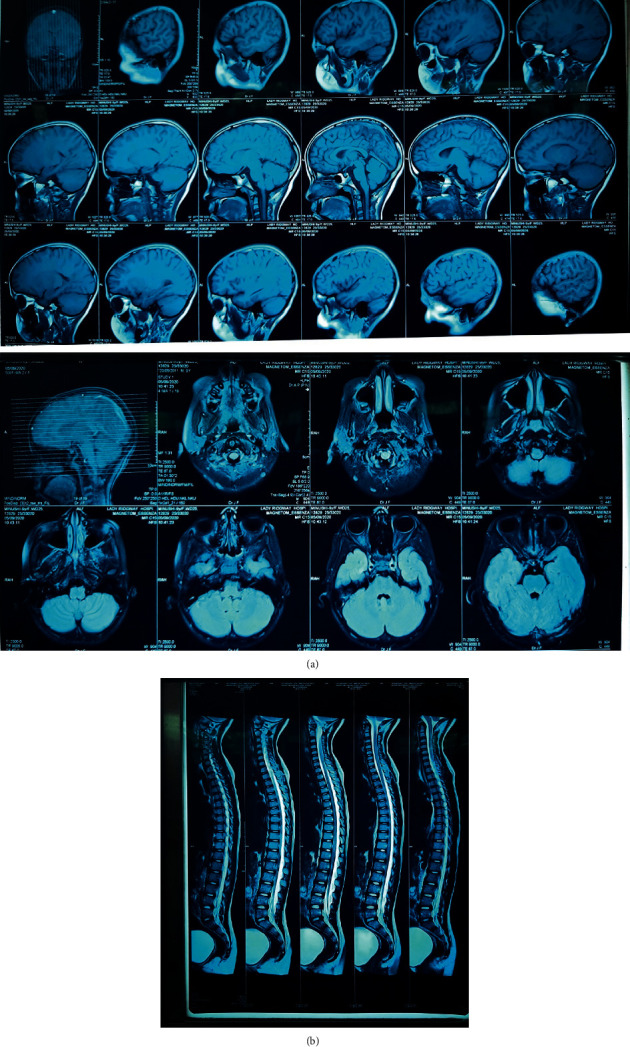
Normal MRI of the (a) brain and optic tract without gadolinium enhancement and (b) spine without postgadolinium enhancement.
